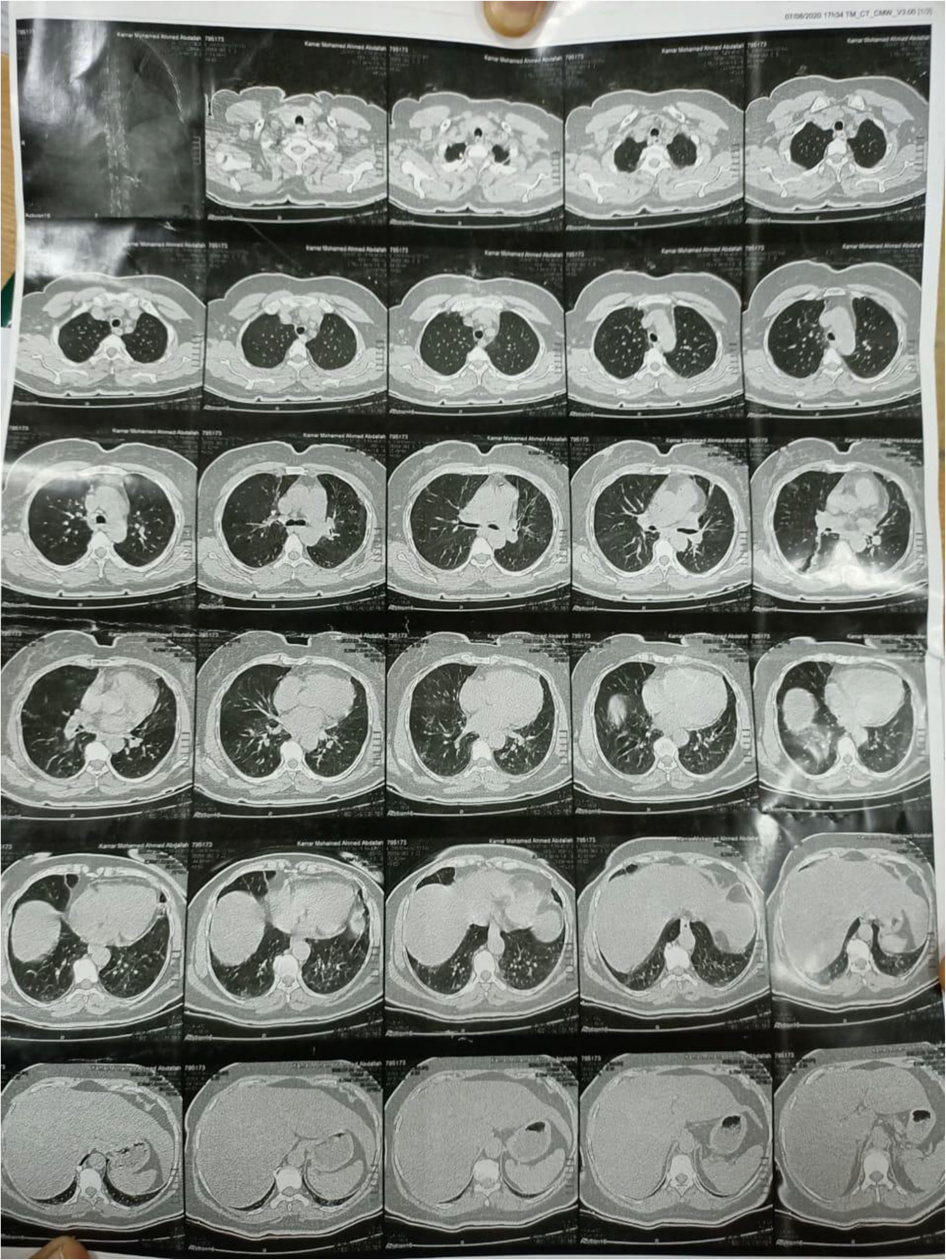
CT chest revealed mosaic attenuation with mainly subplural ground glass opacities with thickened vascular wall as well as septal thickening in keeping with small airways obstruction disease
